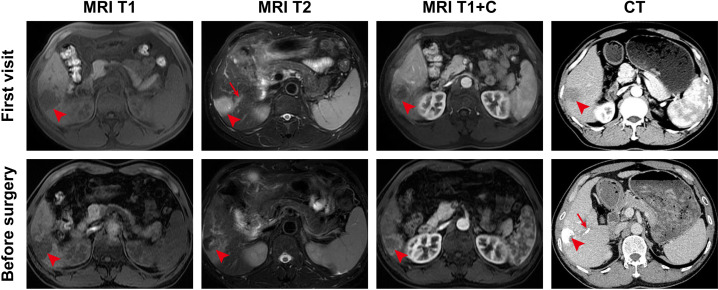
Baseline and post-TACE imaging of the primary hepatic tumor and portal vein tumor thrombus. Magnetic resonance and contrast-enhanced CT images obtained at initial diagnosis and after the first session of transarterial chemoembolization (TACE) before surgery. Panels include T1-weighted, T2-weighted, and contrast-enhanced T1-weighted MRI, as well as contrast-enhanced CT. Arrowheads indicate the hepatic tumor, and arrows indicate the portal vein tumor thrombus. CT, computed tomography; MRI, magnetic resonance imaging.
